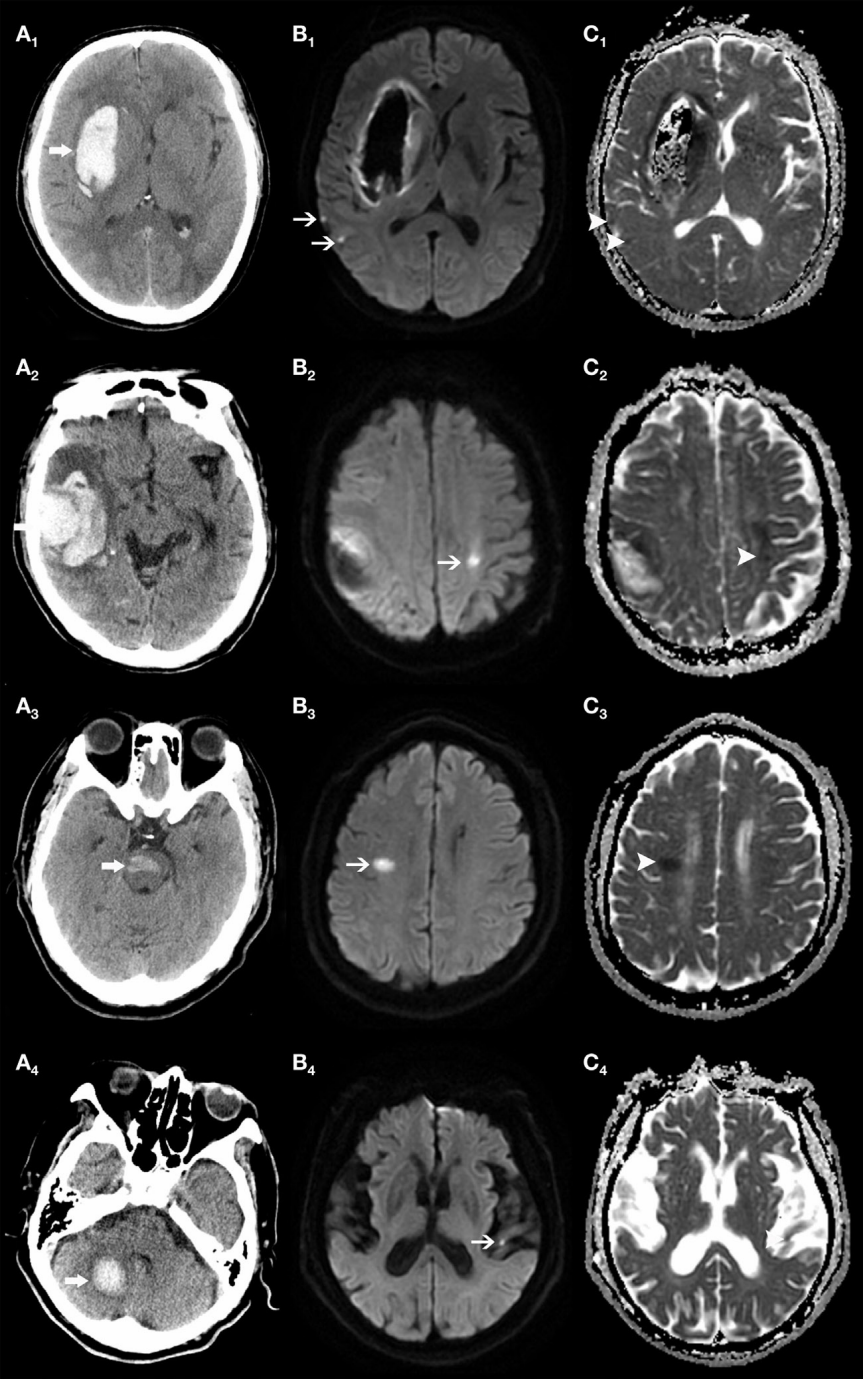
Remote DWI lesions (R-DWILs) are present in patients with bleeding at various sites. The computed tomography shows four patients with intracerebral hemorrhage in right basal ganglia (A_1_), right temporal lobe (A_2_), brainstem (A_3_), and right cerebellum (A_4_), with R-DWILs located at ipsilateral temporal cortex (B_1_, C_1_), contralateral postcentral gyrus (B_2_, C_2_), right centrum semiovale of frontal lobe (B_3_, C_3_), and contralateral temporal cortex (B_4_, C_4_) in diffusion-weighted imaging and ADC, respectively.
